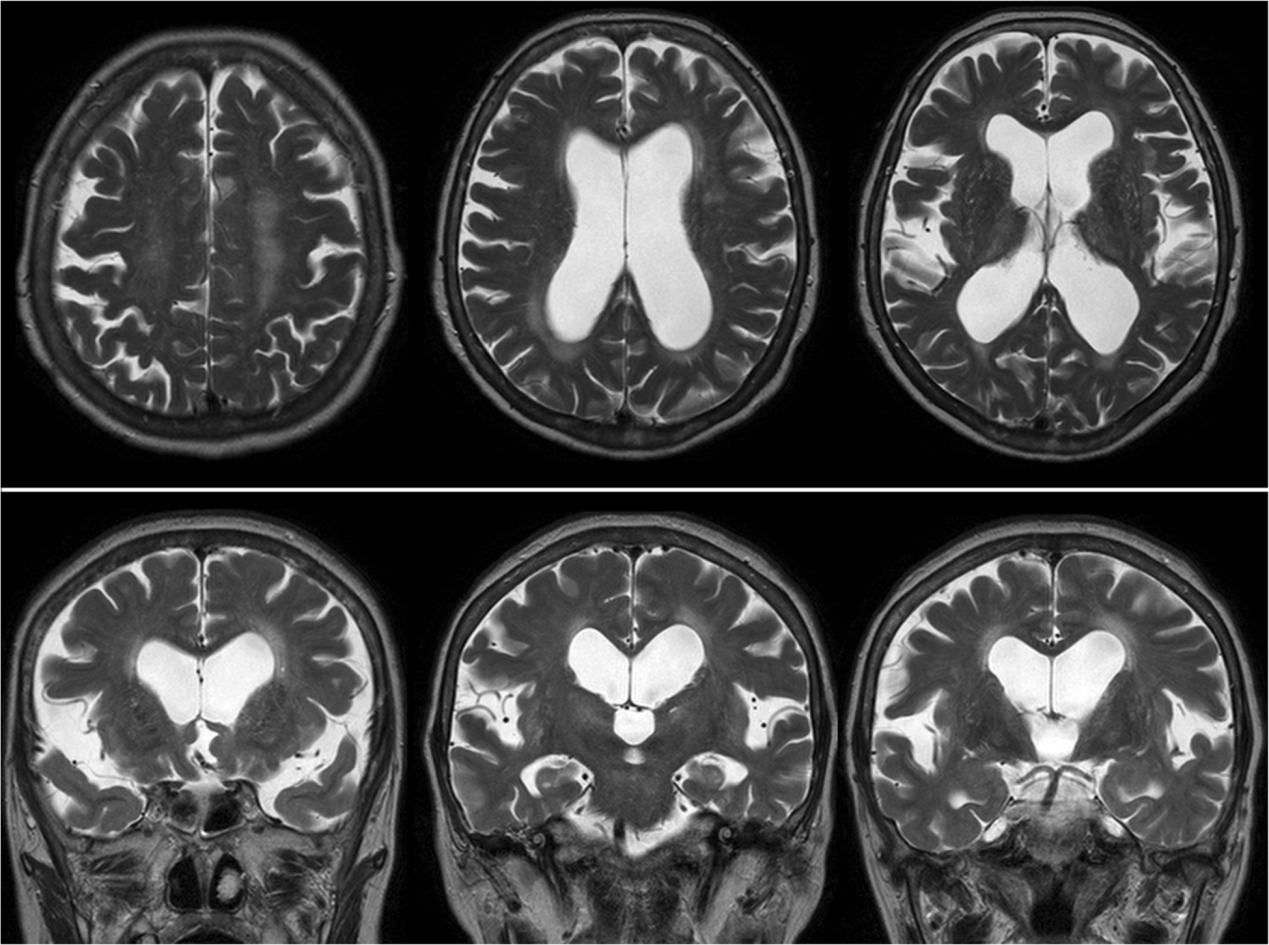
Upper: T2-weighted axial MRI revealed enlarged ventricles. Lower: In T2-weighted coronal MRI also showed enlarged ventricles, however, the major feature of disproportionately enlarged subarachnoid-space was not obvious
